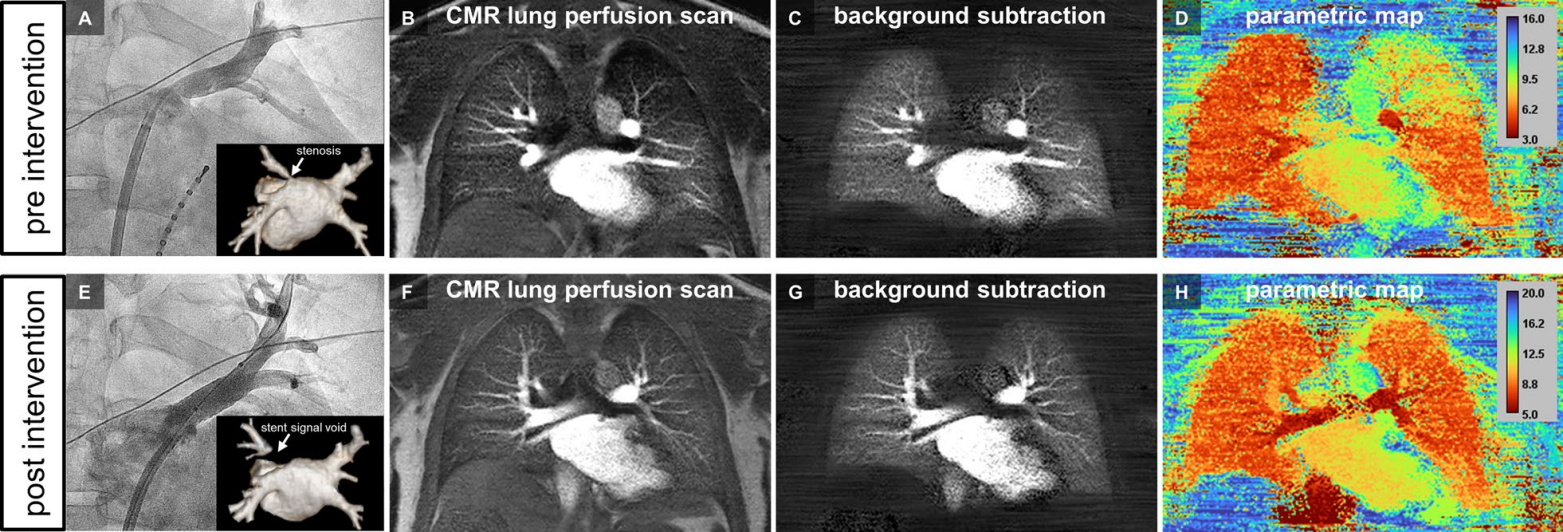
Pre- and post-interventional CMR pulmonary perfusion imaging for assessment of the treatment effect after stenting of a severe ostial left superior pulmonary vein (LSPV) stenosis. Upper row (pre-intervention): Invasive X-ray angiography (A, anterior–posterior projection) and pre-interventional CMR angiography (inlay of A, posterior–anterior projectional view) revealed a ≥ 70% ostial stenosis of the LSPV (white arrow). CMR pulmonary perfusion imaging depicted a perfusion deficit of the left upper lung lobe (B, still frame of original dynamic pulmonary perfusion; C, still frame of dynamic pulmonary perfusion after background stationary tissue subtraction; D, corresponding pseudo-colored parametric map of quantitative CMR pulmonary perfusion analysis with time-to-peak enhancement as the quantitative measure). Bottom row (post-intervention): Invasive X-ray angiography (E, anterior–posterior projection) and post-interventional CMR angiography (inlay of E, posterior–anterior projectional view) after successful angioplasty/stenting of PV stenosis with stent-related signal void (white arrow). On post-interventional CMR pulmonary perfusion imaging at day 1, homogenous perfusion of all lung lobes was observed (F, still frame of original dynamic pulmonary perfusion; G, still frame of dynamic pulmonary perfusion after background stationary tissue subtraction; H, pseudo-colored parametric map of quantitative CMR pulmonary perfusion analysis with time-to-peak enhancement as the quantitative measure; Additional file 2: video file)
